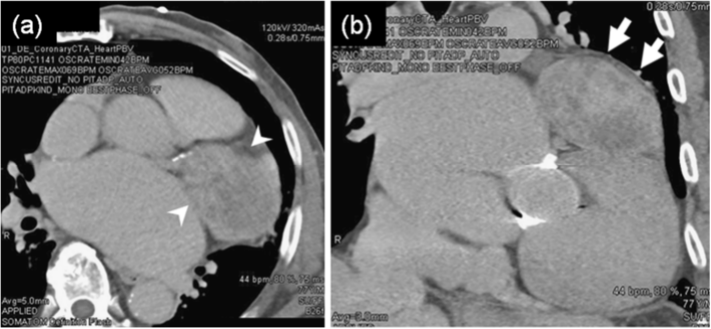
Chest computed tomography (CT). (a) A 77-year-old man presented with an intrapericardial mass. Contrast-enhanced CT demonstrated a huge mass adhered to the left atrial appendage. The mass was not uniform; it lacked calcification and showed non-homogeneous enhancement (arrowheads). (b) Coronal contrast-enhanced CT shows that the mass was covered with pericardium (arrows).
